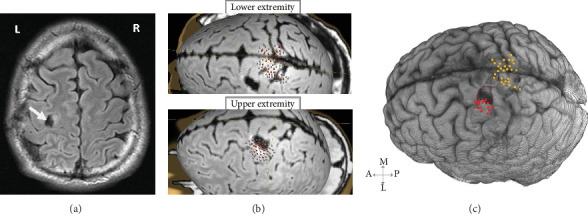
(a) Postoperative axial FLAIR MRI 1 year later in axial view showing resection cavity. L, left; R, right. (b) Follow-up nTMS 1 year later superimposed on FLAIR MRI. Red dots represent points with MEPs for upper and lower extremity. (c) 3D rendering of the patient's MRI 1 year later with the nTMS points coregistered. Red dots represent the upper extremity, and yellow dots represent the lower extremity. A, anterior; P, posterior; M, medial; L, lateral.
